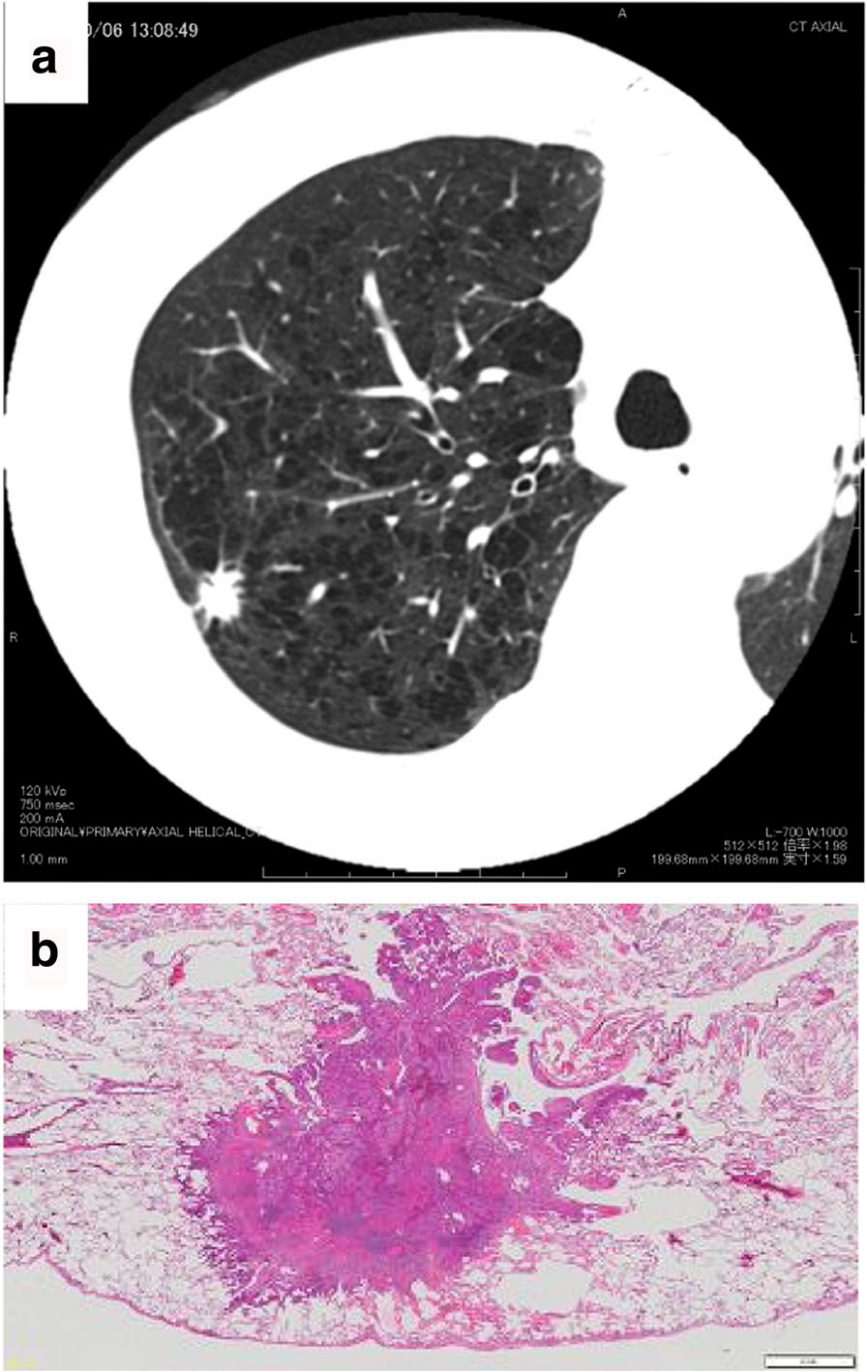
a Chest thin-slice computed tomography (TSCT) showing a 1.1-cm solid nodule in the right upper lobe. b Pathologic features of the nodule, showing adenocarcinoma (macroscopic image)
